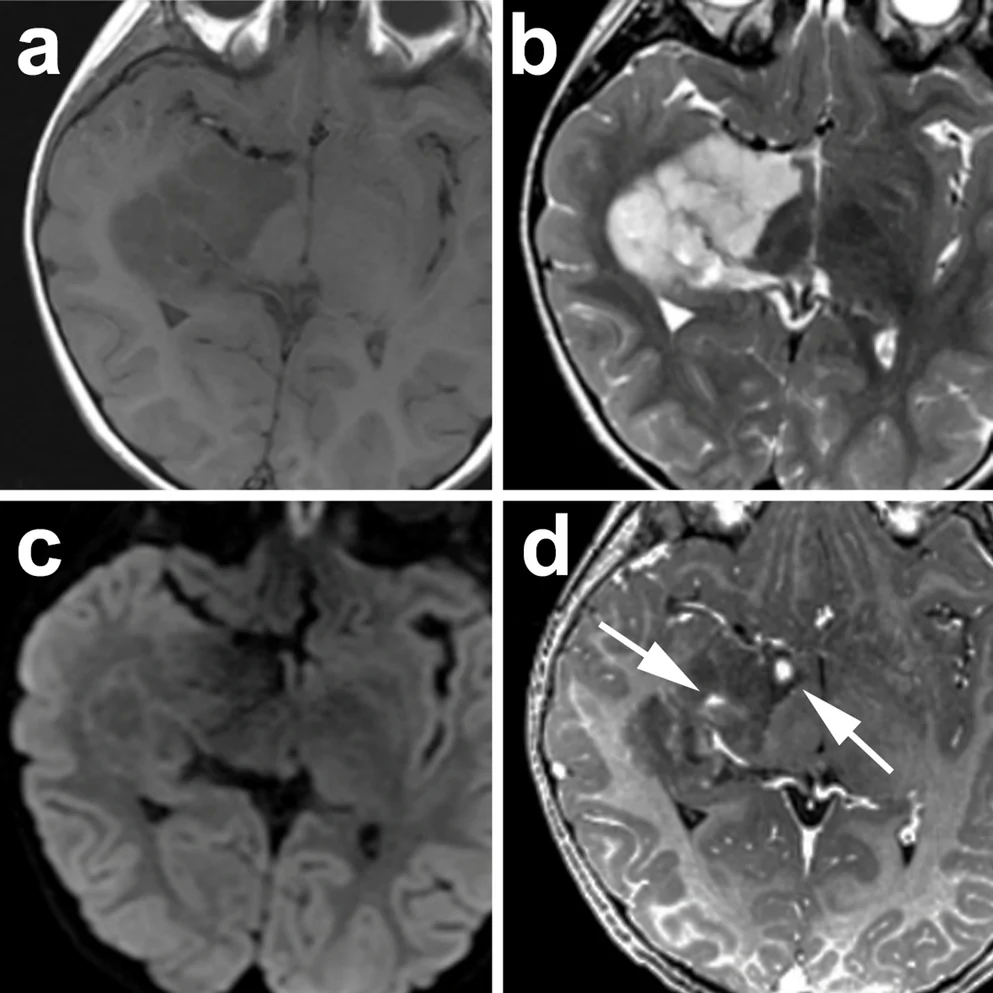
A 4-year-old boy with dysembryoplastic neuroepithelial tumor presenting with absence seizures. A well-circumscribed mass with a cystic-like appearance is seen in the right mesial temporal lobe, showing low signal intensity on the T1-weighted image (a) and high signal intensity on the T2-weighted image (b). No obvious hyperintensity is observed on the diffusion-weighted images (c). The contrast-enhanced T1-weighted image (d) demonstrates nodular enhancement (arrows)
